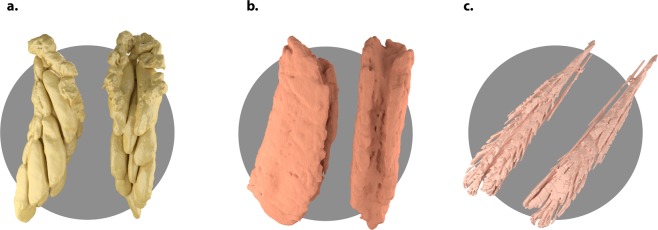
Internal organs of *Pseudochorthippus parallelus* measured using x-ray computed tomography. (a) The initial egg load was measured as the volume of egg carrying ovaries. (b) In males, we measured fat body volume (this tissue was almost inexistent in females at this developmental stage). (c) Femora muscle volume (extensor and flexor together) was measured as a proxy for investment in muscle tissue in both sexes.
